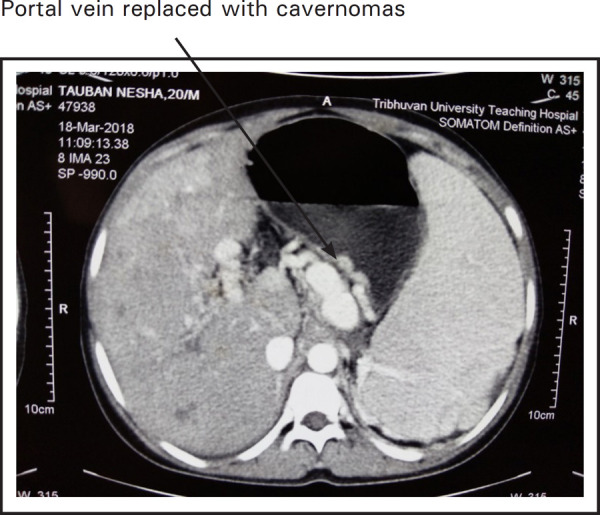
CT scan showing cavernomas and varices.
